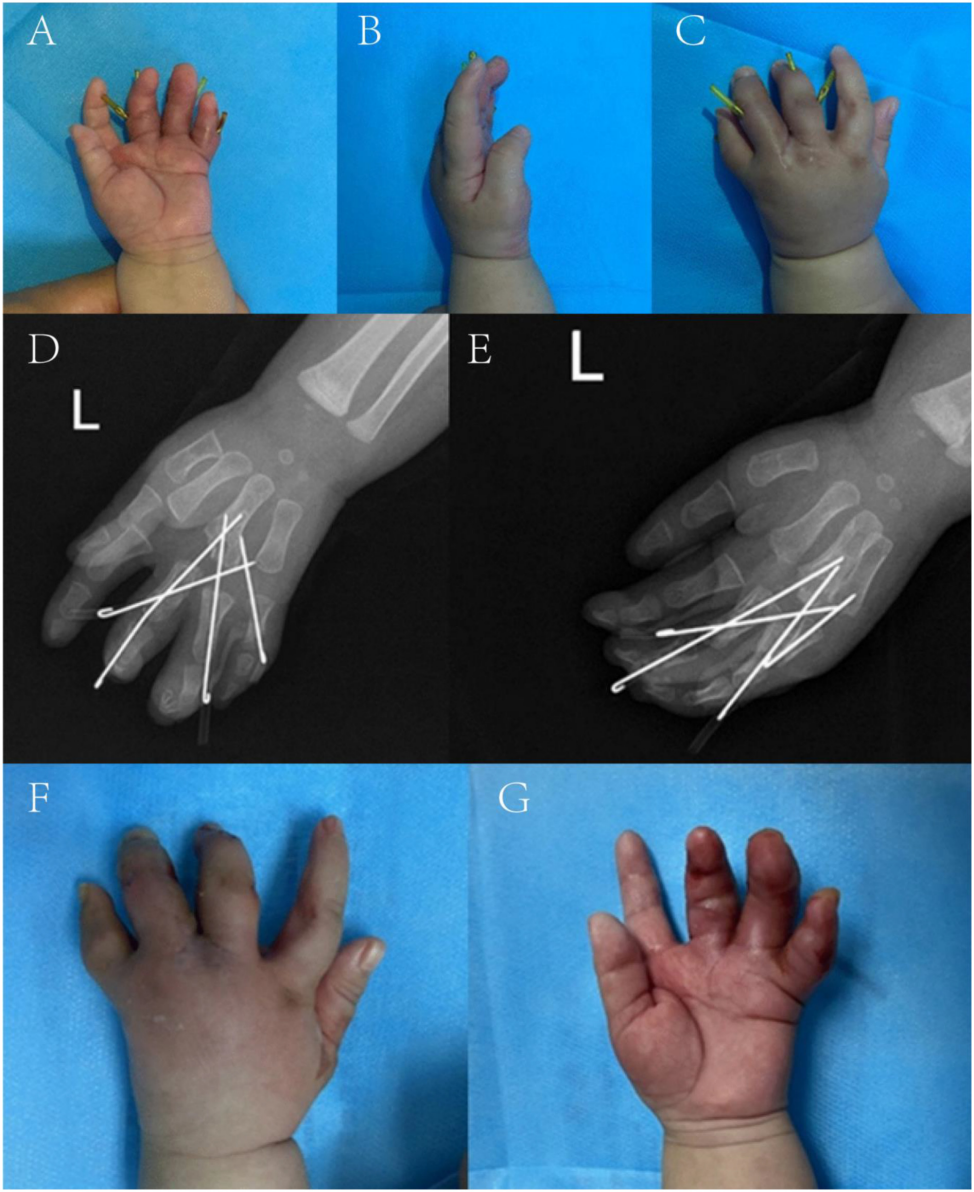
Follow-up pictures at five weeks after the operation. (A–C) Appearance photographs. (D,E) x-ray films. (F,G) Appearance after the removal of Kirschner wire.
